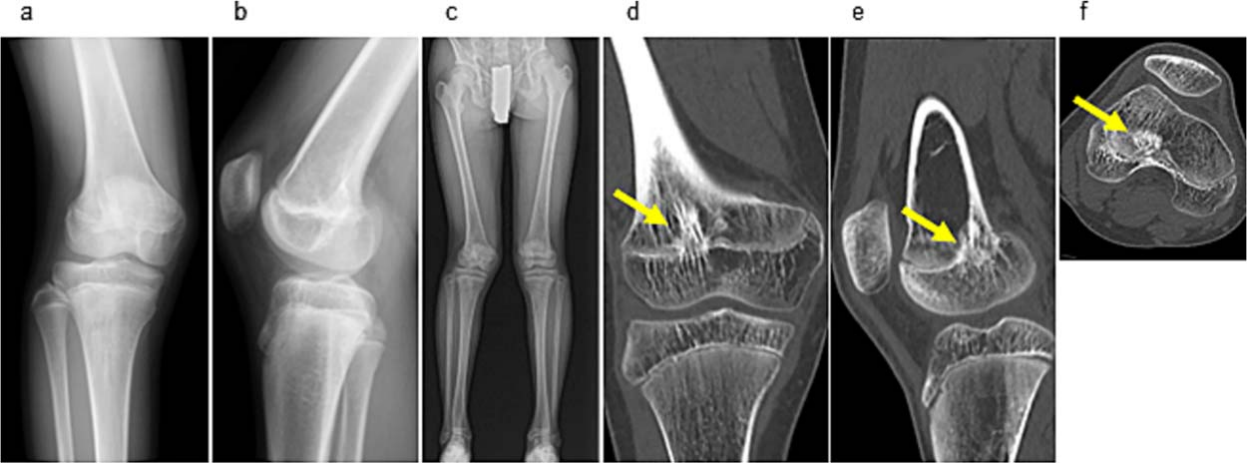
Preoperative radiographic and CT findings. (a) Anteroposterior radiograph of the right knee. (b) Lateral radiograph of the right knee. (c) Full-length standing anteroposterior radiograph of the lower extremities showing valgus deformity (femorotibial angle: right, 165°; left, 172°). (d) Coronal CT image. (e) Sagittal CT image. (f) Axial CT image showing early epiphyseal closure of the distal femur caused by a physeal bar (arrow), located adjacent to the posterior cortical bone.
